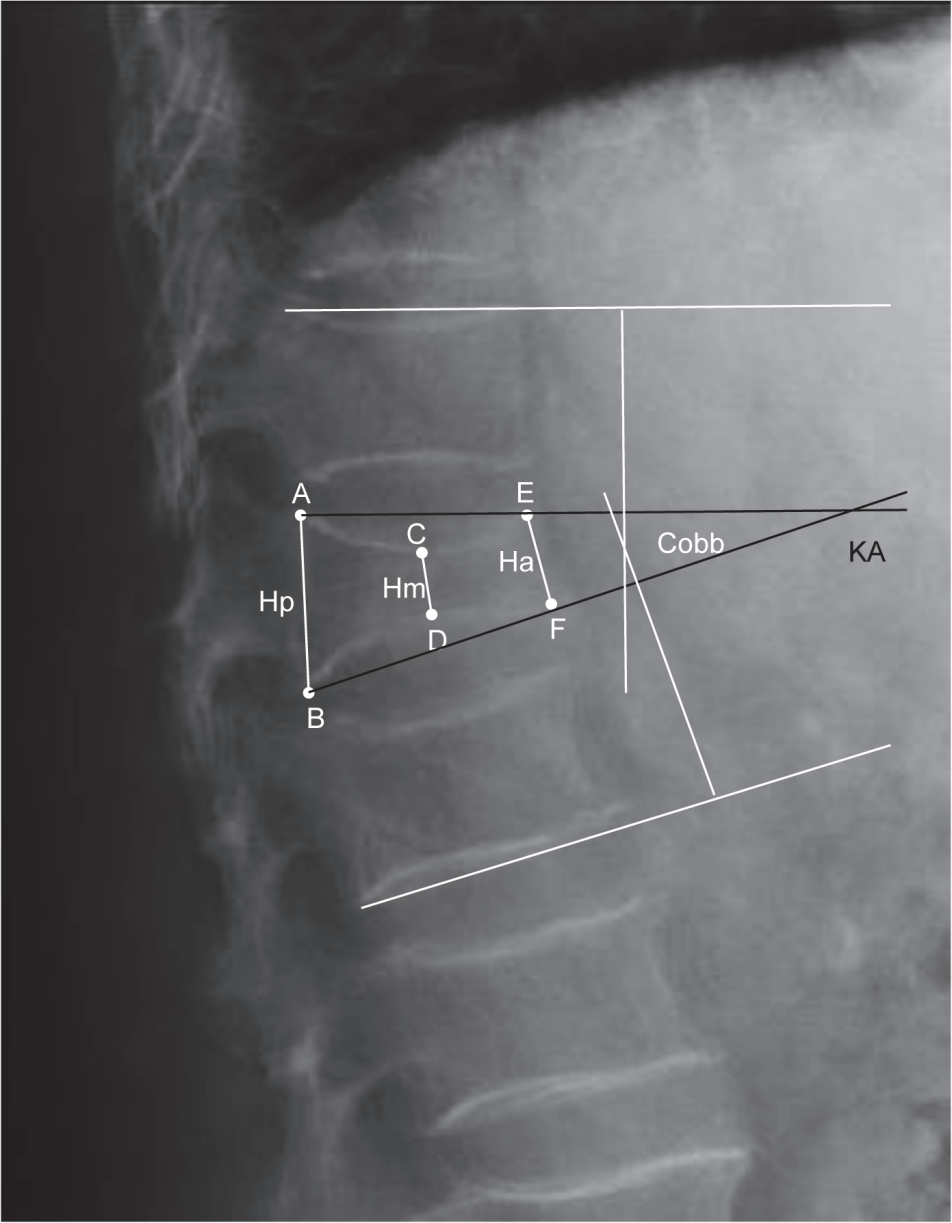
Radiographic measurement of vertebral body height in sagittal alignment using 6 defined points: A and B on the most dorsal-superior and—inferior endplate margins, E and F correspond to the most anterior-superior and—inferior margins, C and D are on the midpoint of a perpendicular line drawn from A to E and B to F on the superior and inferior vertebral endplates. Cobb- and vertebra body kyphotic angle.
